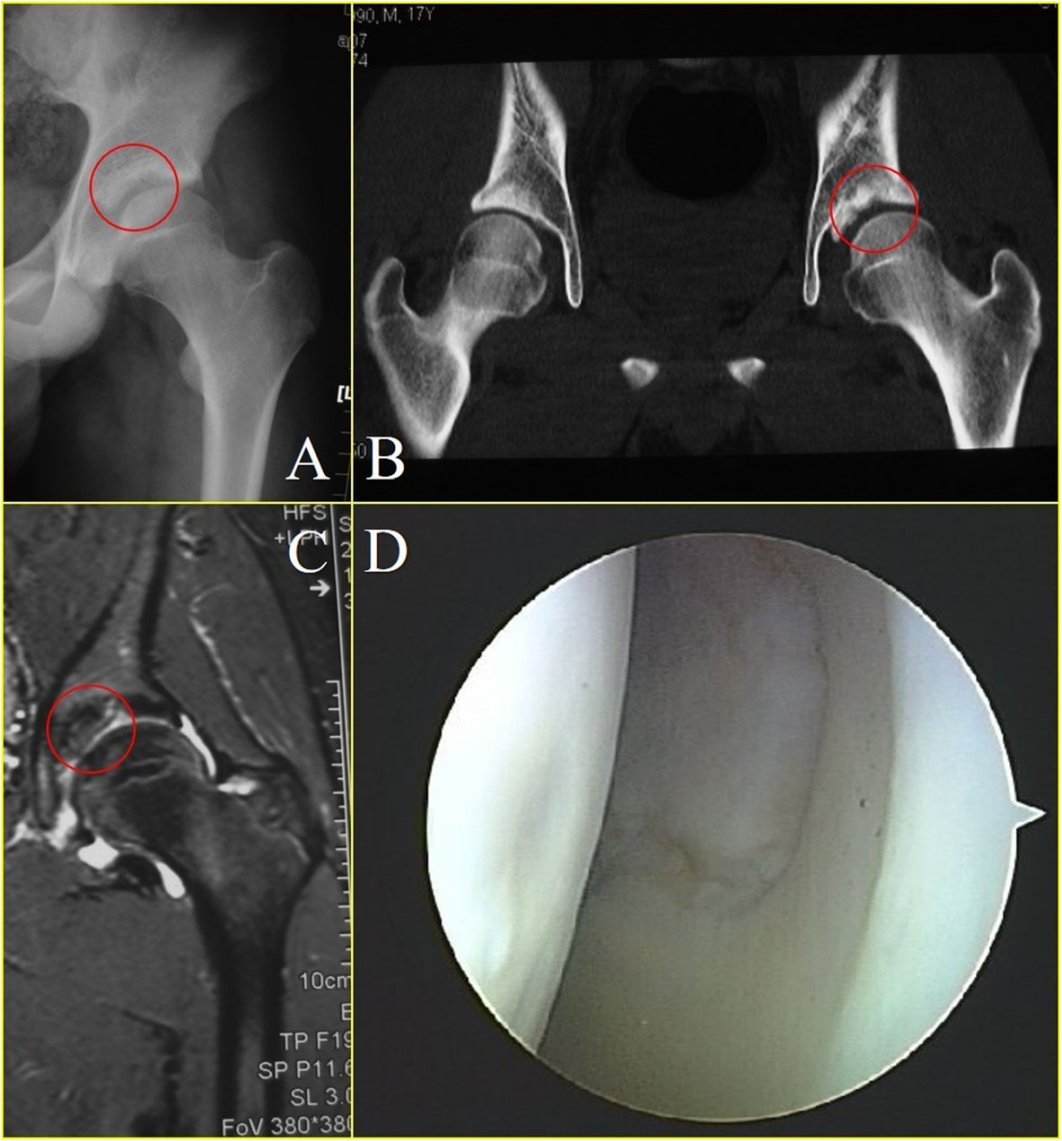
An atypical radiological appearance identified on plain radiograph of three patients with wedged clump type loose bodies. Red circle indicate loose bodies. (a: anteroposterior plain radiograph. b: CT scan. c: MRI. d: arthroscopic view.)
